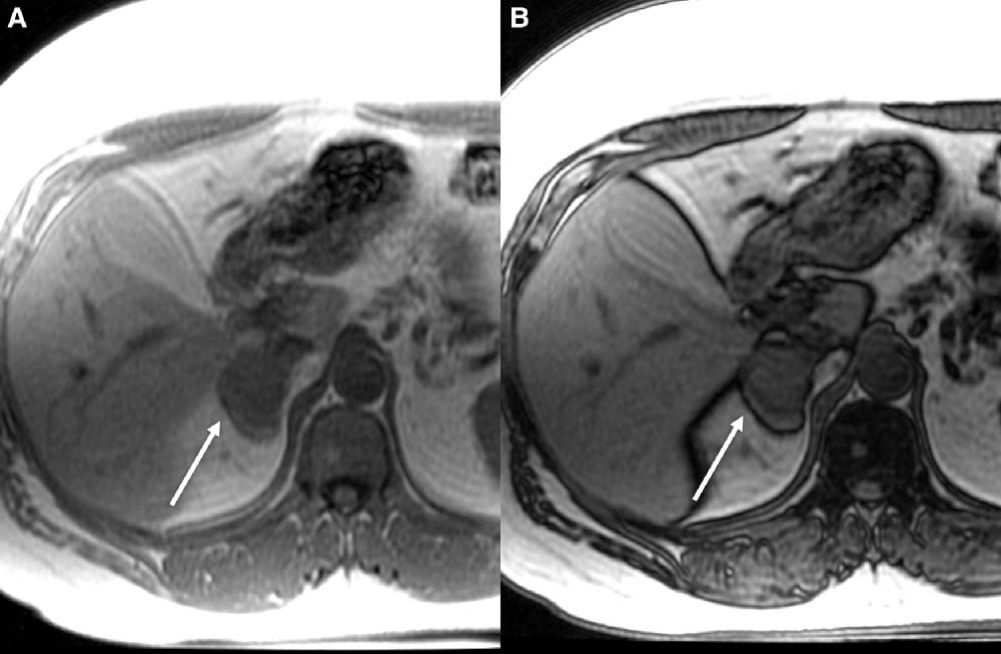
Axial chemical shift MRI demonstrates minimal reduction in signal from in-phase (A) to out-of-phase phase imaging (B), consistent with a lipid-poor indeterminate right adrenal mass.
